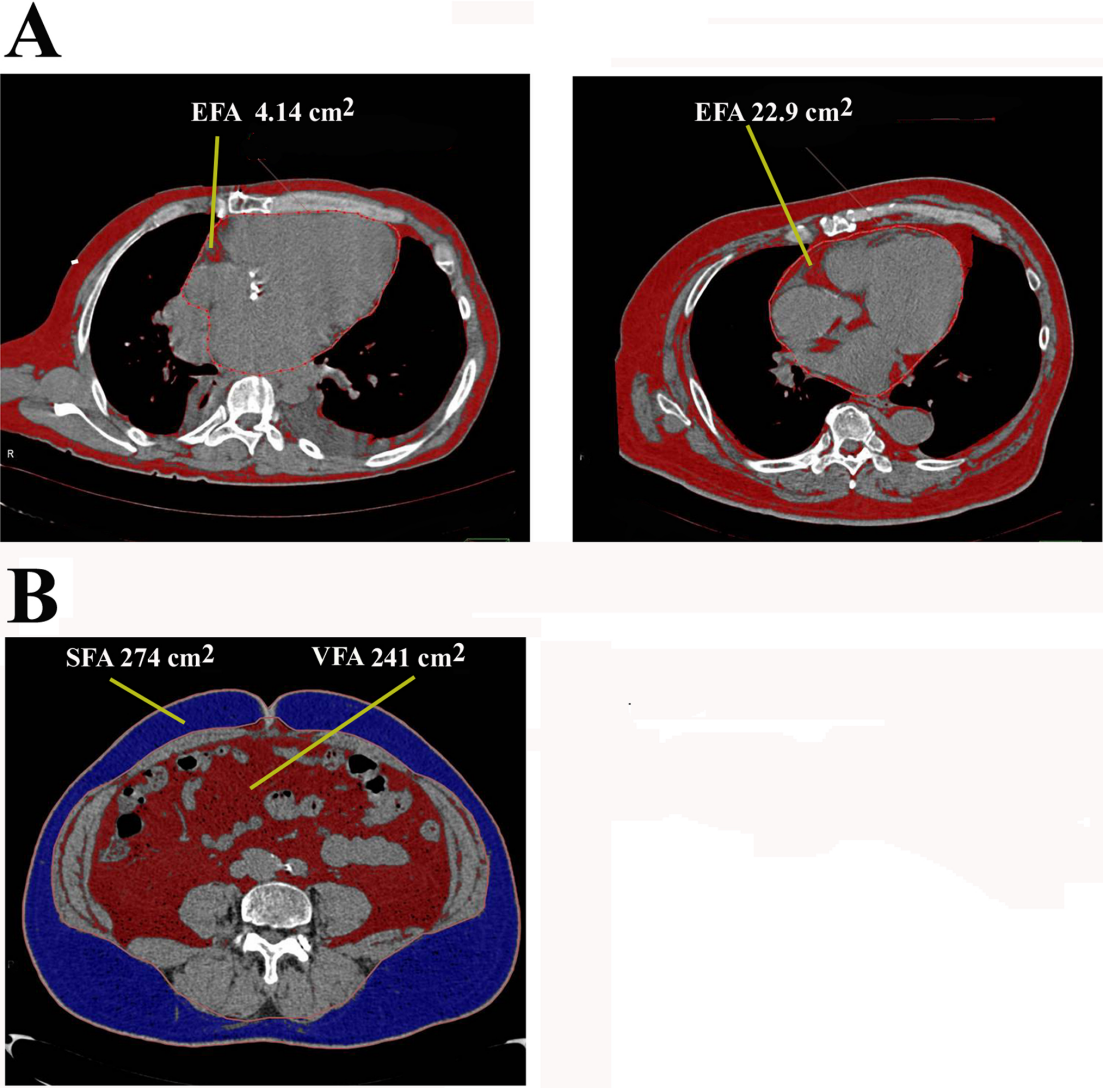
Measurement of fat area by CT scan. A: Measurement of epicardial fat area (EFA). Two representative cases are shown. The image is shown at the position where the heart becomes a long axis image with respect to the apex of the heart in the axial section image with ROI. The heart surrounded by ROI and the epicardial fat area (EFA, cm^2^) was measured by a semi-automated method with CT value defined as -150 to -50 HU. Fat is identified as brown color. EFA is estimated as 4.14 cm^2^ and 22.9 cm^2^, respectively. B: Measurement of subcutaneous and visceral fat area. The areas of abdominal visceral fat (VFA, cm^2^, brown color), and subcutaneous fat (SFA, cm^2^, blue color) were determined at the cross-sectional image of the umbilicus. Fat was measured by a semi-automated method with CT value defined as -150 to -50 HU.
